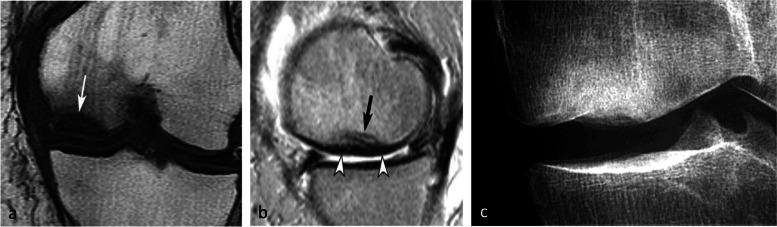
Early subchondral insufficiency fracture with osteonecrosis (SIF-ON) and collapse. a T1-weighted MR image shows an area of low signal intensity in the inferior pole of the medial condyle (arrow). b T2-weighted image (b) shows an immediate subchondral low signal intensity area longer than 14 mm (arrowheads), suggestive of early SIF-ON. Note also several fissure lines away from the surface (arrow), which is also a risk factor for an evolution towards a SIF-ON. c A few years later, a radiograph shows an irregular collapsed articular surface, confirming the initial suspicion of SIF-ON
